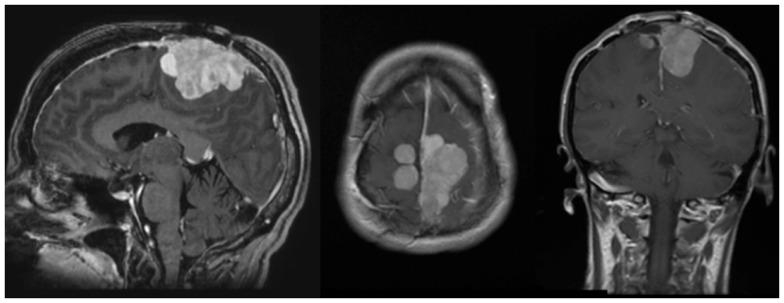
Pre-operative MRI. MRI (l–r, sagittal, axial, coronal) demonstrates an avidly enhancing, complex falcine meningioma involving both sides of the superior sagittal sinus.
